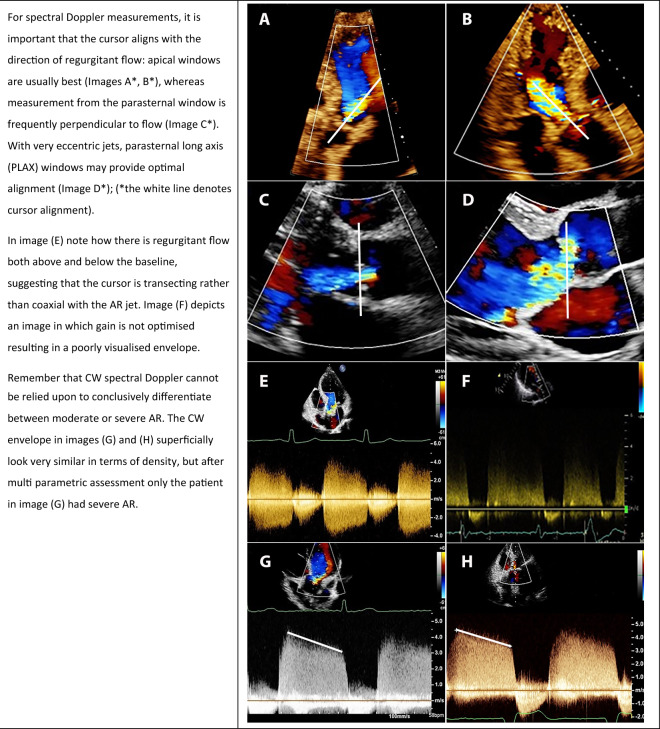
Spectral Doppler: how best to approach assessment of aortic regurgitation
